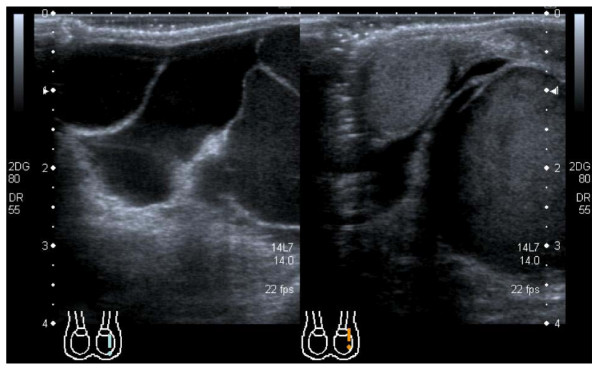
The scrotal ultrasound scan demonstrates the multilocular cystic structure expected in scrotal lymphangioma.
